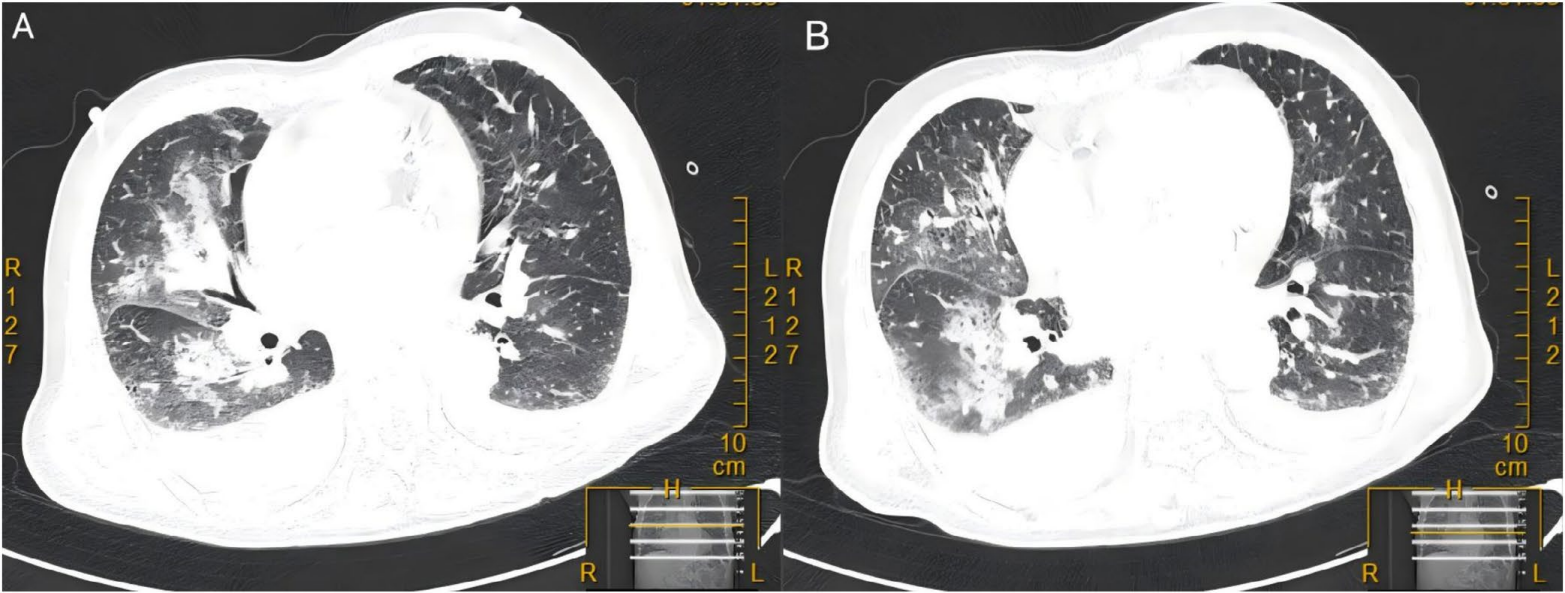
Chest CT showed bilateral pulmonary edema with pleural effusion (A and B).
